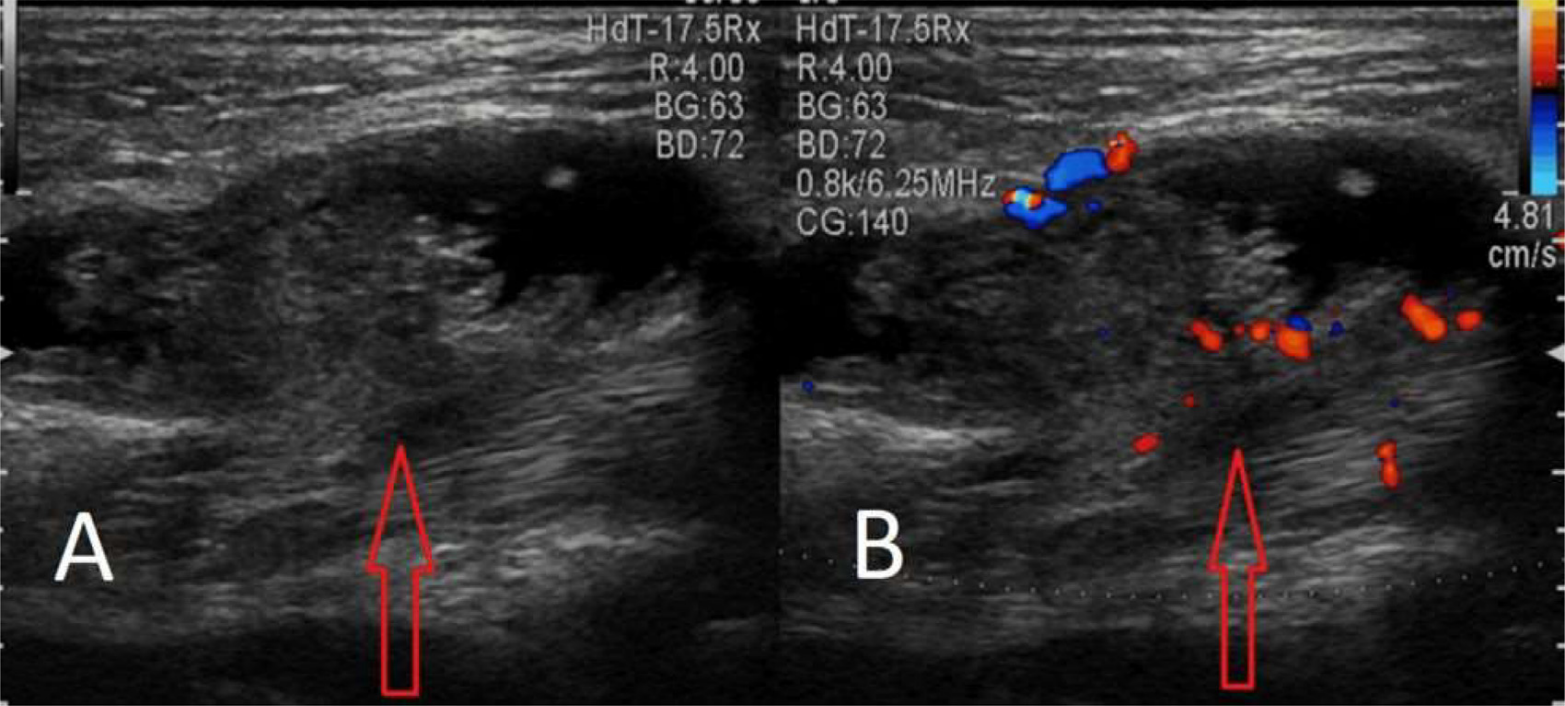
Ultrasound grey scale B mode image of right popliteal fossa showing cystic lesion with internal echoes suggestive of morrant baker cyst (red arrows). A-Grey scale B mode image, B-Grey scale B mode image with color doppler.
